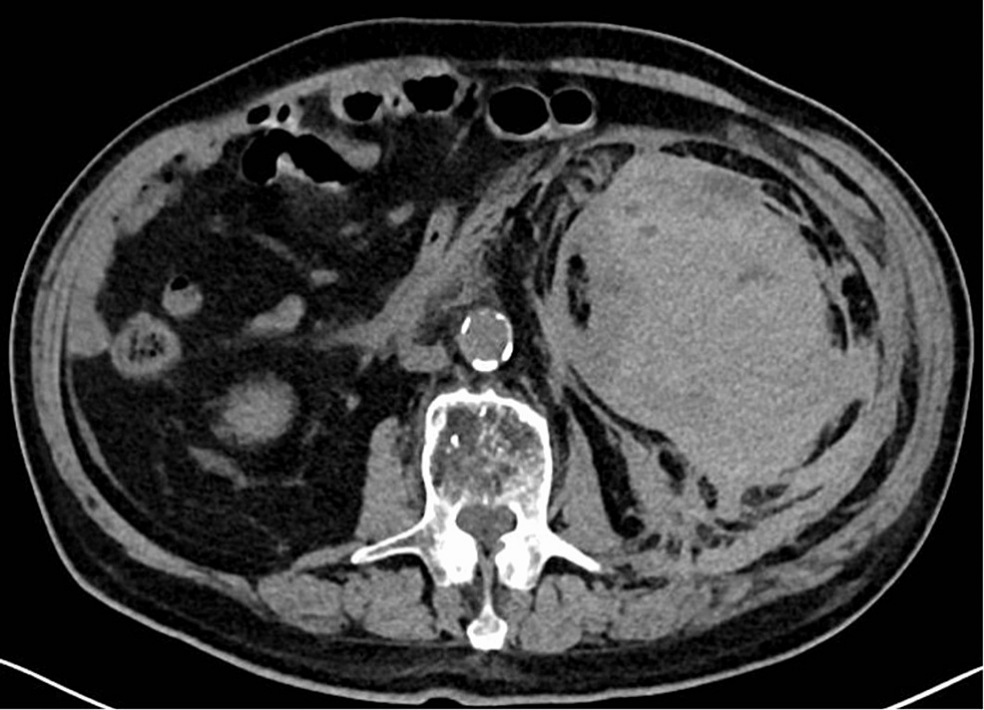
CT image of left perinephric hematoma. CT: computed tomography.
